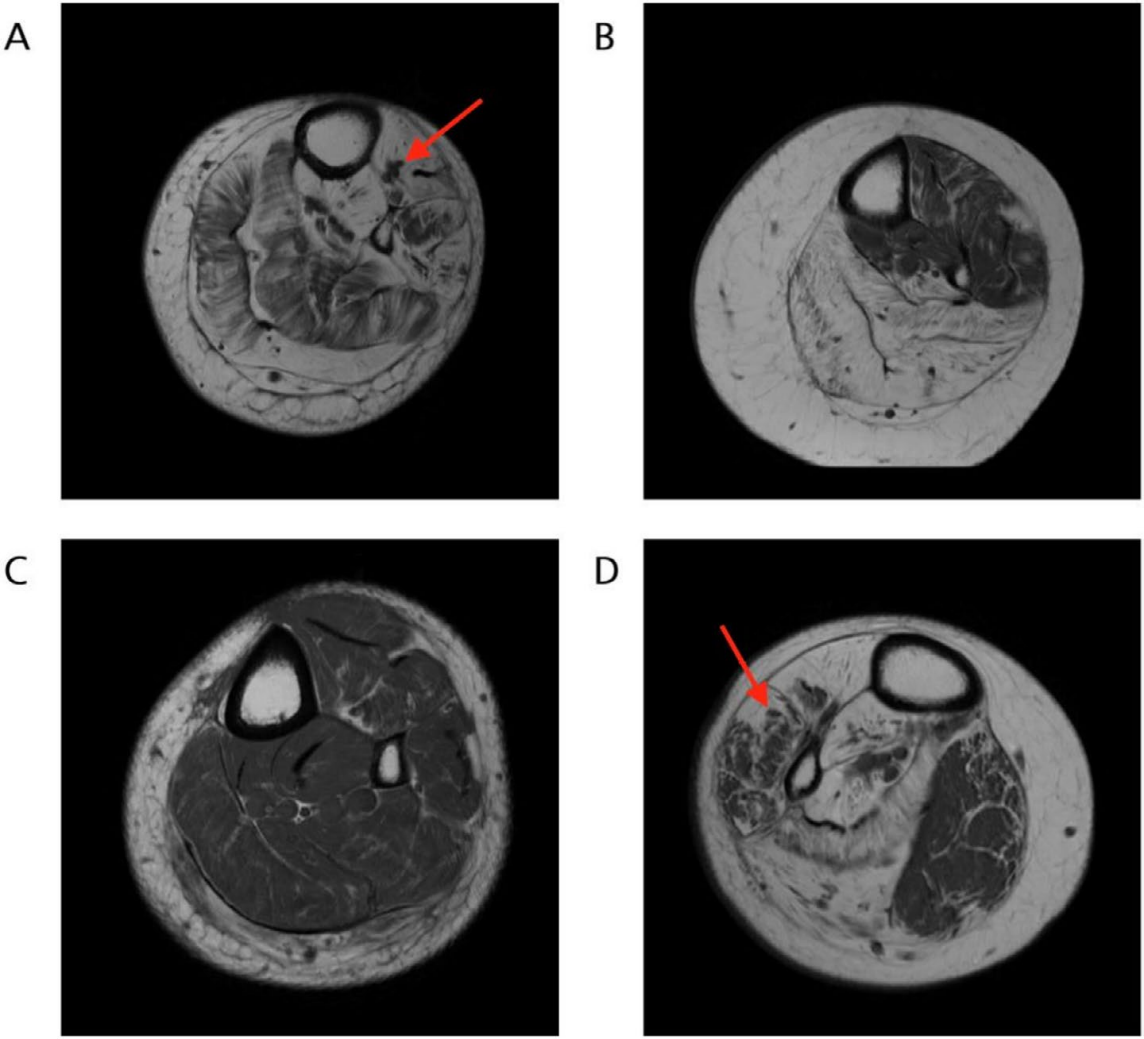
T1‐weighted muscle MRI of less‐affected legs of participants at the level of mid‐calf. (A) P03 demonstrating diffuse fatty infiltration throughout the muscle with neuromuscular “islands” of sparing in tibialis anterior (arrow). (B) P04—extensive fatty replacement in the posterior compartment with patchy fatty infiltration in the anterior compartment. (C) P05—relatively normal muscle with little fatty infiltration. (D) P06—extensive fatty infiltration and neuromuscular islands (arrow) throughout anterior compartment with relative sparing of medial head of gastrocnemius.
